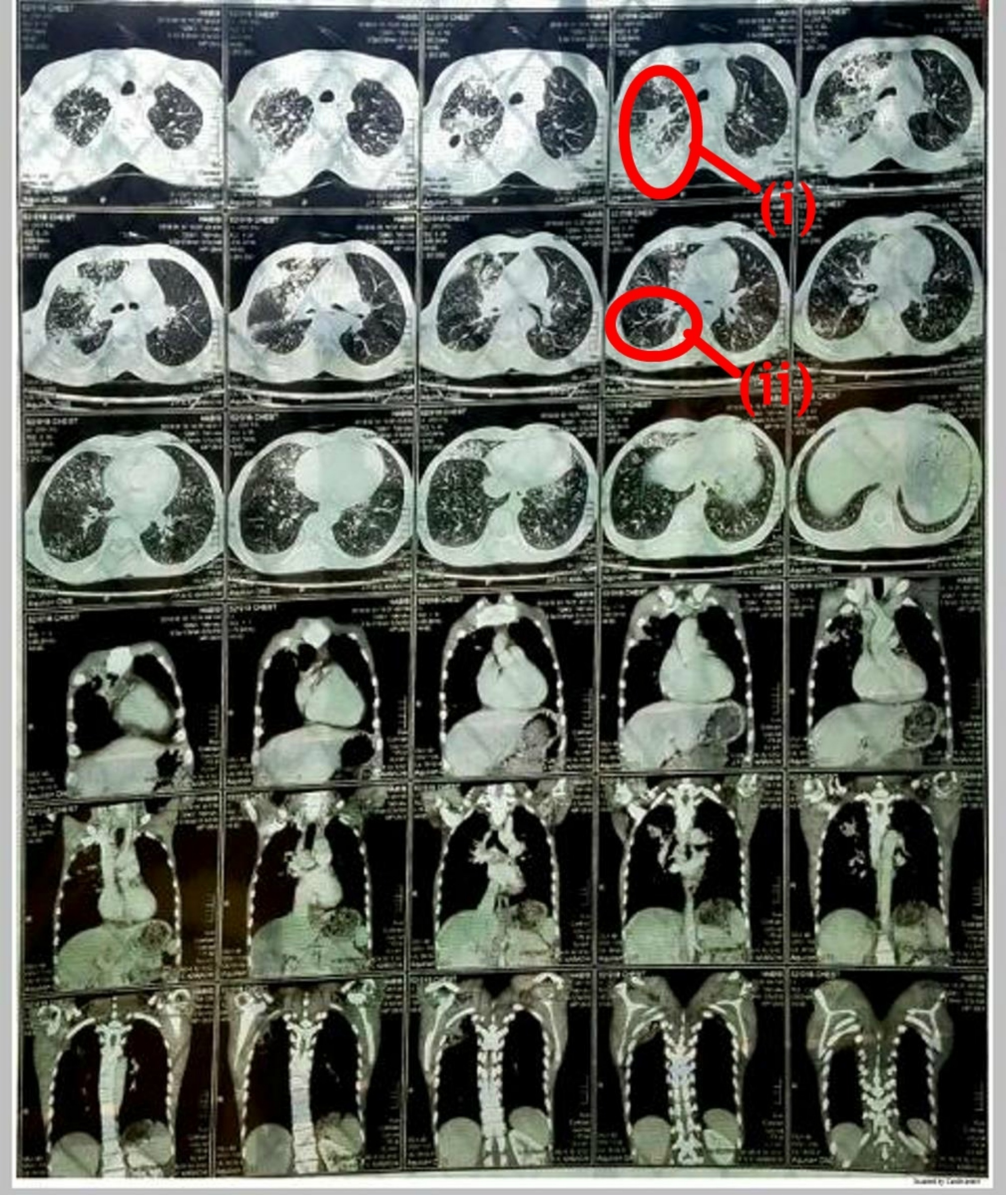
CT scan chest: (i) Multiple fluffy alveolar infiltrates; (ii) tree-in-bud appearance CT: computed tomography
